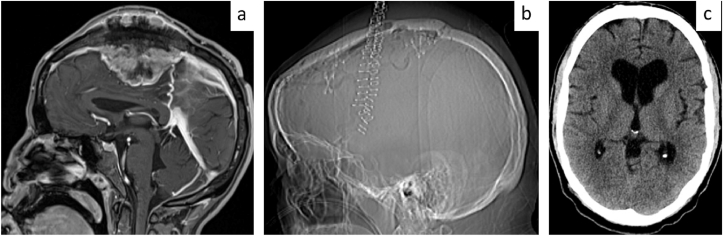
Representative Case 2: CSF fistula after meningioma resection – primary LPS implantation. 3a. Contrast-enhanced T1-weighted cranial MRI demonstrating a large exophytically growing osteomeningioma. 3b. Cranial CT scout image obtained after tumor resection and primary closure, including duraplasty and cranioplasty with palacos and microplate fixation. 3c. Axial cranial CT scan obtained four weeks postoperatively showing ventricular enlargement consistent with postoperative hydrocephalus requiring CSF diversion.
